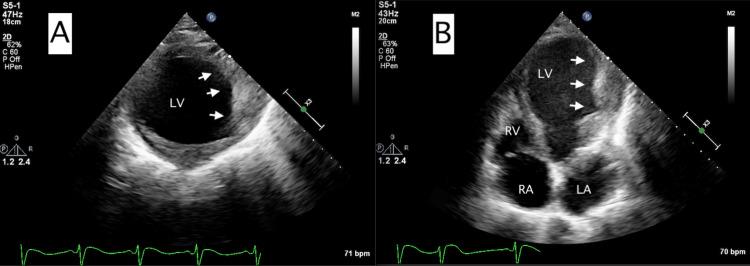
Transthoracic echocardiogram with thrombus formation in pseudoaneurysm There is severe left ventricular dilation with concentric remodeling of the left ventricle. Anterolateral pseudoaneurysm with an echogenic material suggestive of thrombus formation. A: Left ventricle short axis view with thrombus formation in the pseudoaneurysm (white arrows). B: Four-chamber view with thrombus formation in the pseudoaneurysm (white arrows). LV: left ventricle, LA: left atrium, RV: right ventricle, RA: right atrium.
